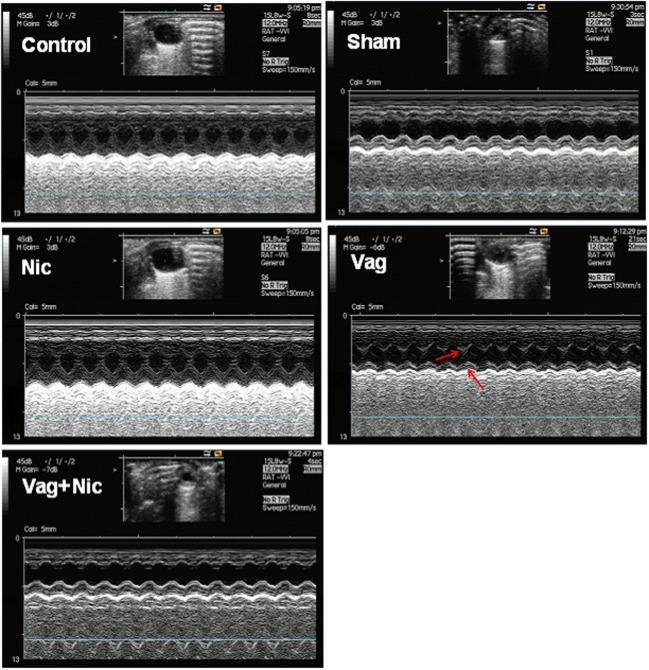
Representative M-type cardiac ultrasound image on day 14. It was shown in the figures that the left ventricle movement of Sham, Vag, and Vag + Nic was weaker than that of control and Nic. Arrows indicated that anterior and posterior ventricular wall motion was not synchronized.
